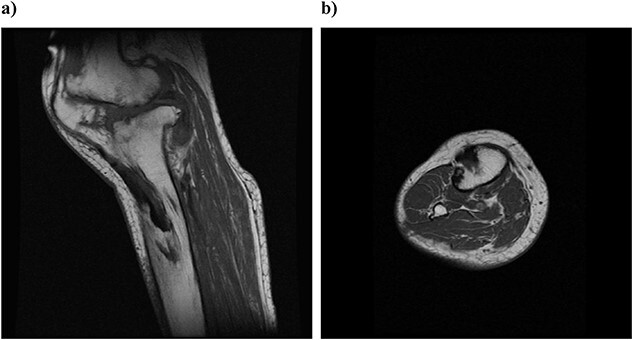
Preoperative simple magnetic resonance imaging: (a) lateral side and (b) axial morphism. T1- and T2-weighted images continuous from the patellar tendon attached to the patella both show continuity from the low signal area to the inside of the tibial bone marrow.
